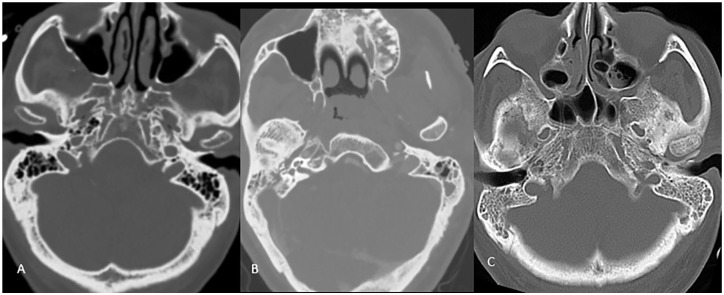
Mastoid opacification grades. Axial CT scan images demonstrate normally
